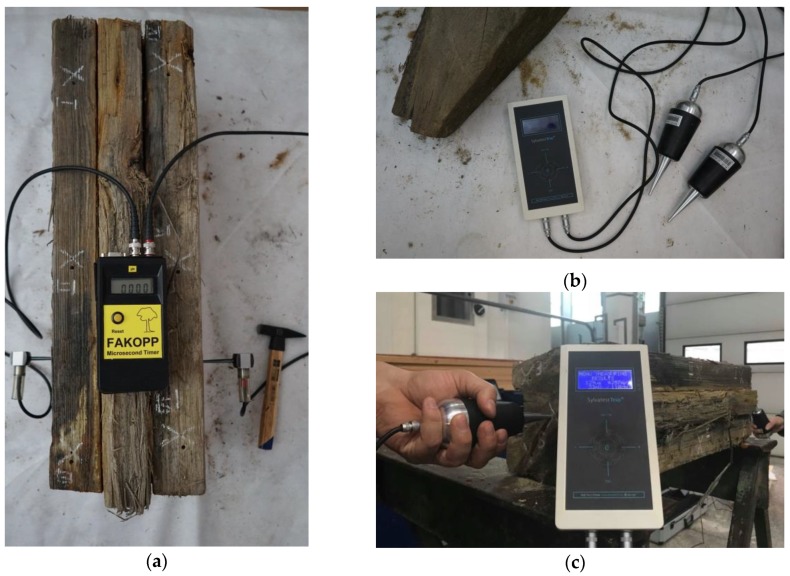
Devices for testing by acoustic method: (a) Fakopp Microsecond Timer using stress wave; (b) Sylvatest Trio device using ultrasonic wave; (c) Sylvatest Trio device during test.
